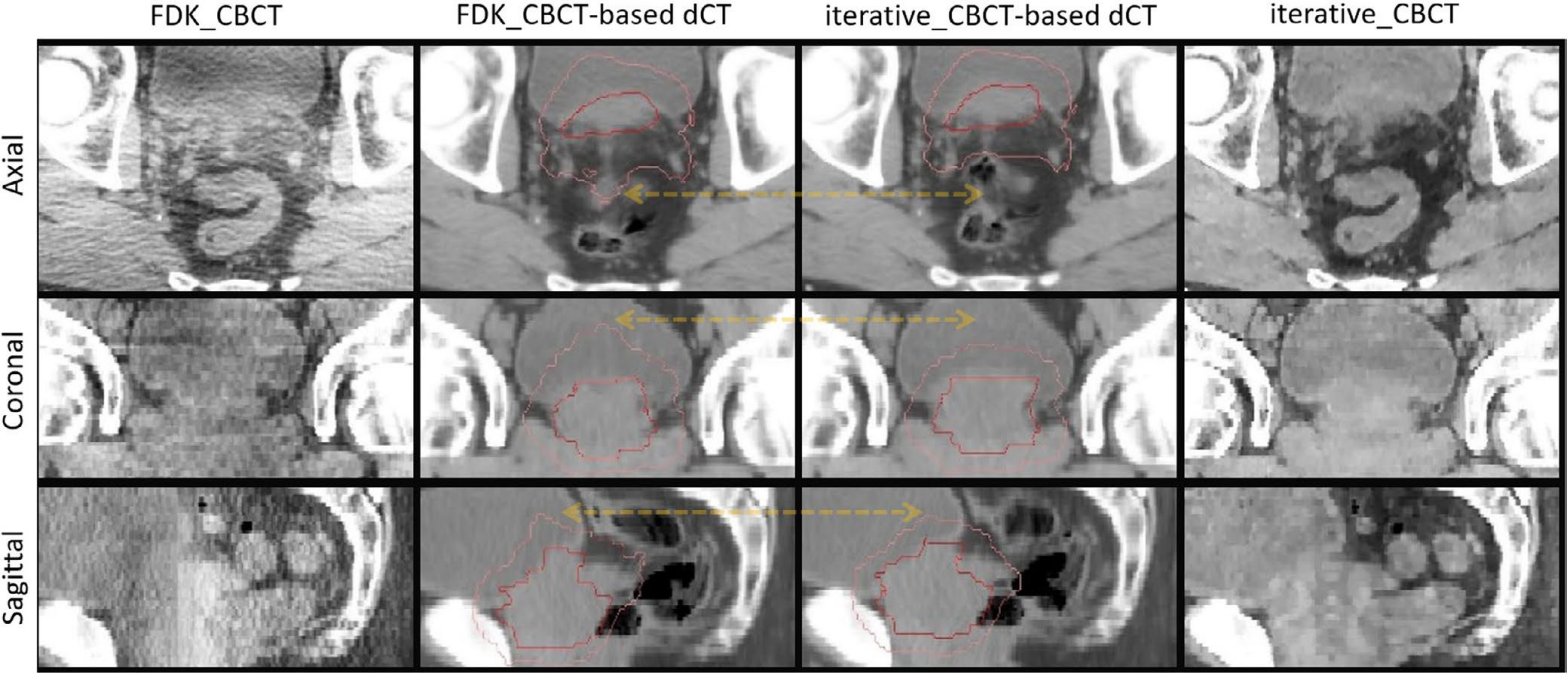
Axial (top row), coronal (middle row), and sagittal (lower row) views of FDK‐CBCT (left column) and iterative‐CBCT (right column) and associated deformed CT (dCT) registered from plan‐CT. Red arrows indicate image variations. HU# [‐300 300].
